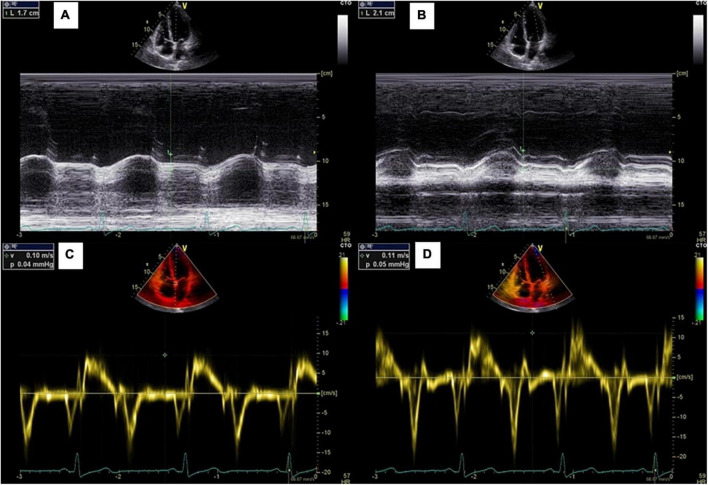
Measurements of mitral annular plane systolic excursion. (A,B) Septal and lateral MAPSE measured by M mode echocardiography; (C,D) Septal and lateral S’ measured by pulsed-wave tissue Doppler.
